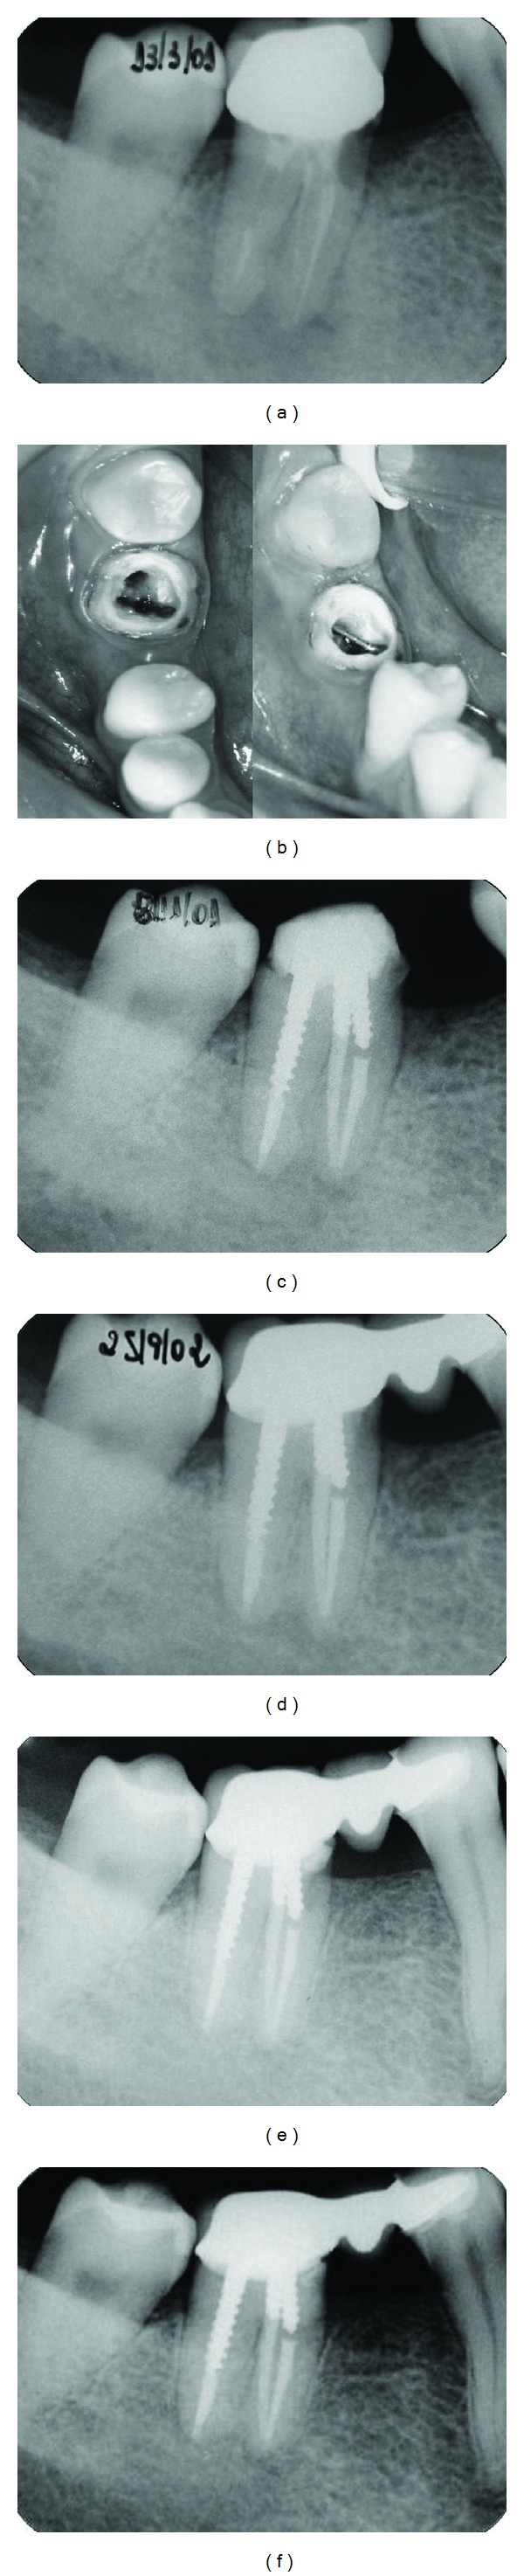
(a) The radiographic evaluation of case 1. (b) Clinical evaluation of the case. (c) Radiographic evaluation after perforation sealing 10 months later, 3 years later (d), 6 years later (e), and 11 years later (f).
